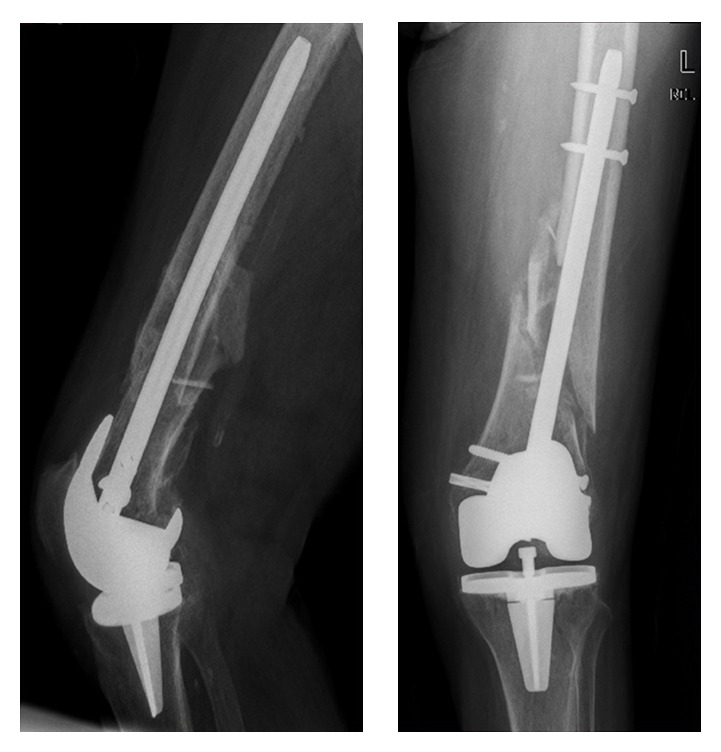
Five months of postoperative AP and lateral left knee X-rays showing signs of bone healing.
